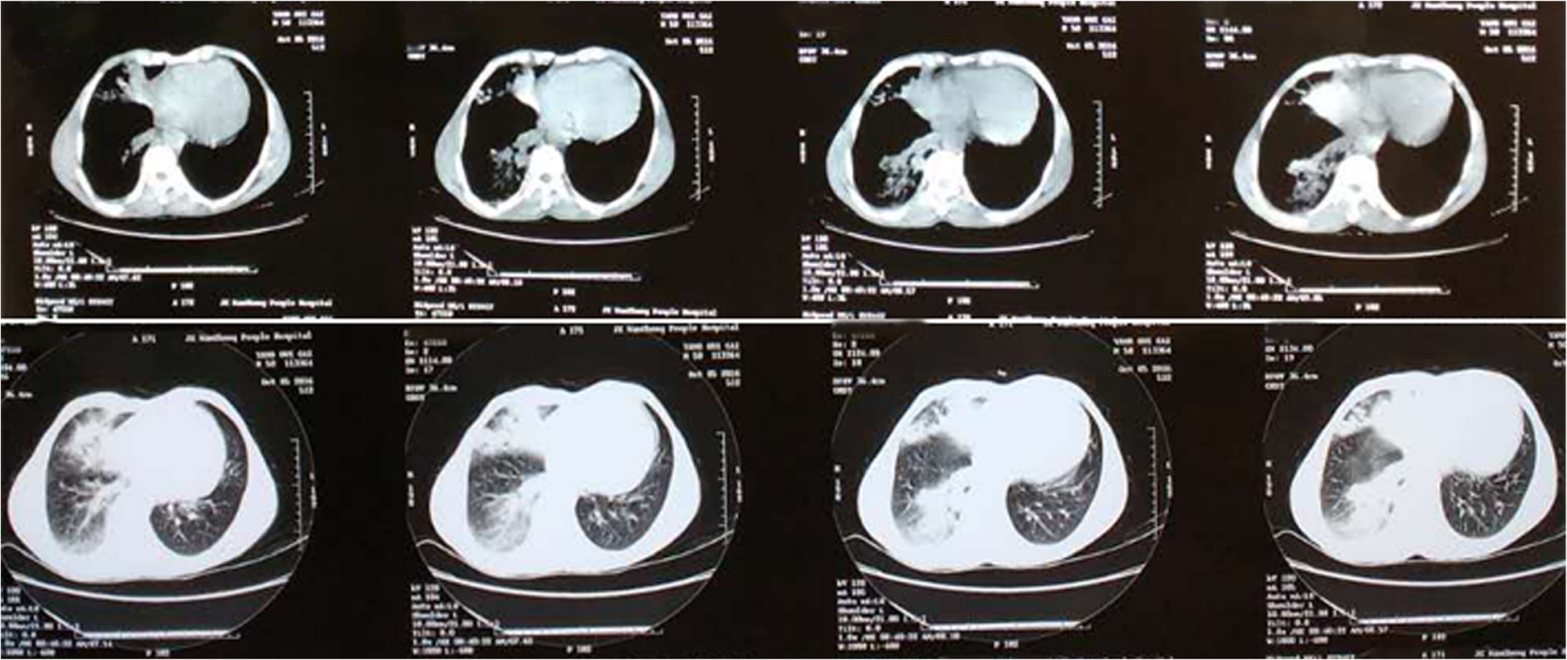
The local hospital chest CT (Oct. 01) showed patchy opacities and a spot-like high density shadow in the right basement of the lower lobe and the right middle lobe
